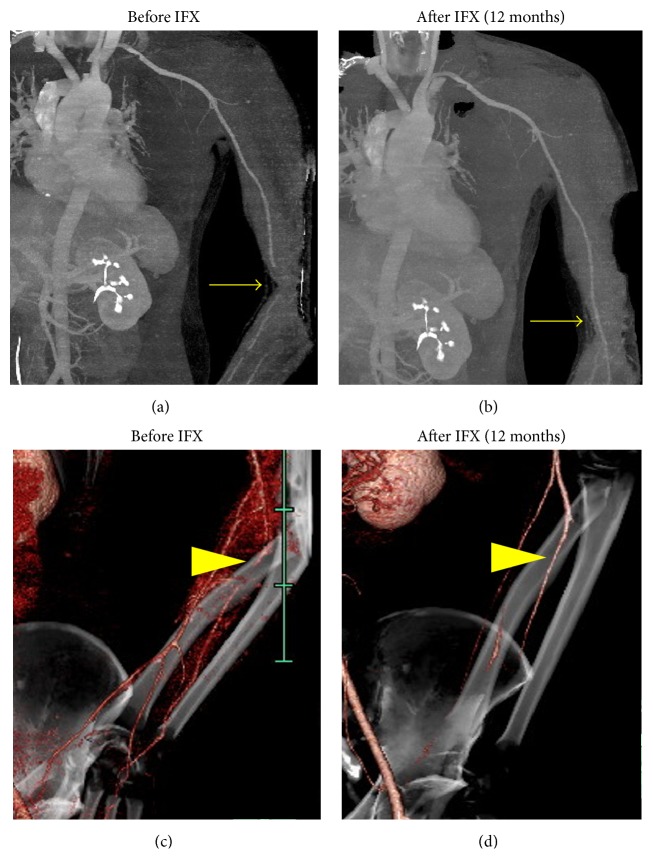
Computed Tomography Angiography showed severe stenosis of left brachial (a) and radial (c) arteries before infliximab (IFX) induction. After IFX therapy, the artery stenosis was immediately resolved and sustained clinical remission ((b) and (d) at 12 months since IFX was introduced).
